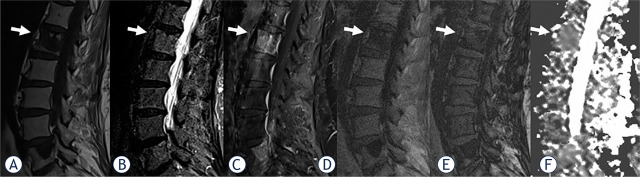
A 75-year-old woman with breast cancer and five focal malignant vertebral bone marrow lesions (VBMLs), the most prominent in the L1 body (arrows) which we chose to analyze. Abnormal signal intensity change is evident on sagittal T1-weighted (A), short tau inversion recovery (STIR), (B), contrast-enhanced fat-satureted T1-weighted (C) in-phase T1-weighted (D), and out-of-phase T1-weighted (E). Apparent diffusion coefficient (ADC) (F) value is 0.99 x 10^−3^ mm^2^/s. The signal intensity ratio (SIR) value is calculated as 1.07. which indicates the malignant lesion. Sci suggested malignant lesions.
